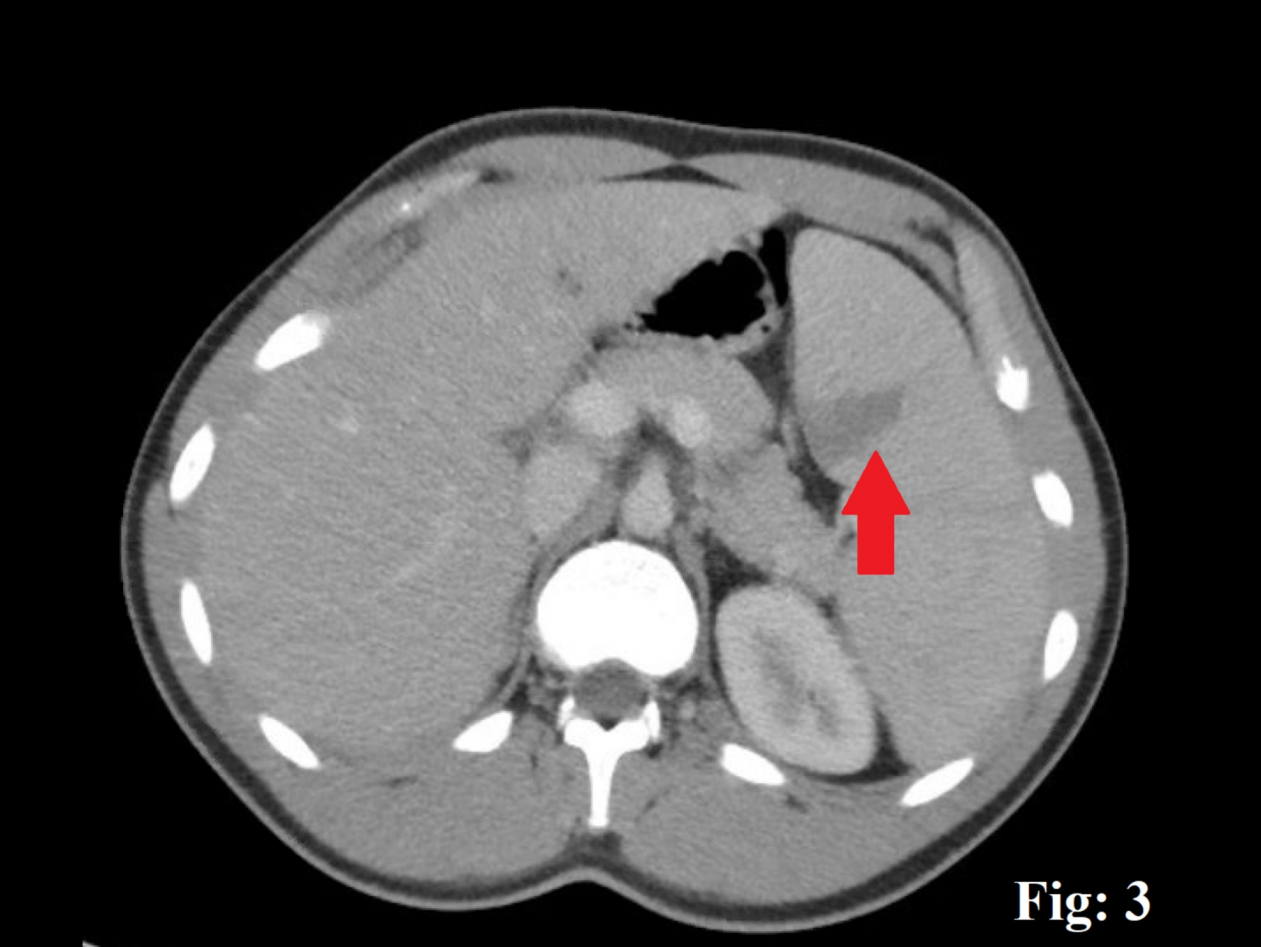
A contrast-enhanced computed tomography scan of the abdomen, the red arrow pointing a wedge-shaped splenic infarct.
